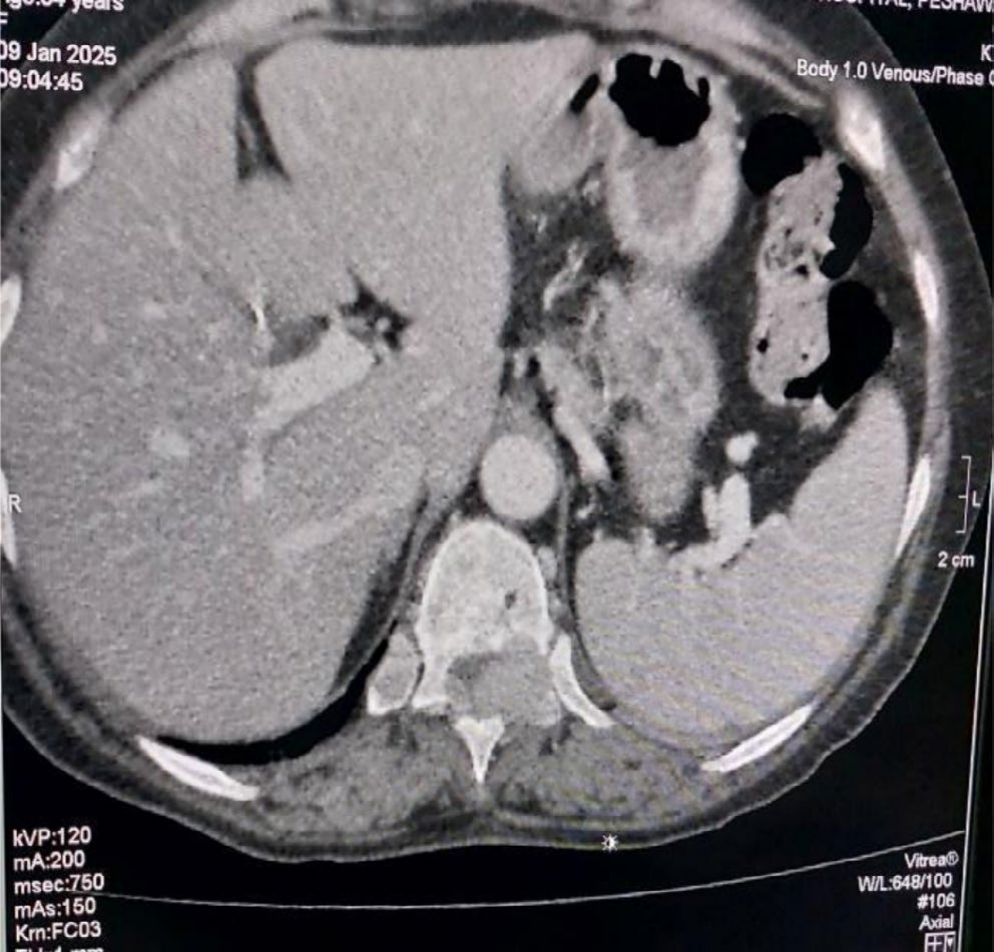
Axial view of CT abdomen and pelvis at the thoracic level showing an osteolytic lesion in one of the ribs.
